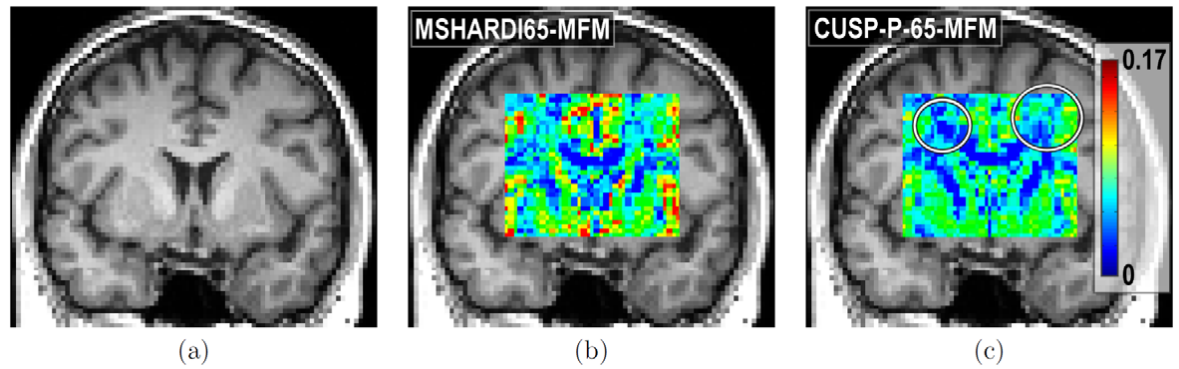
Comparison of CUSP and multi-shell HARDI via residual bootstrapping. (a) T1-weighted image showing the anatomy. (b) Standard deviation of the maximum FA when using MSHARDI-65-MFM. (c) Standard deviation of the maximum FA when using CUSP-65-MFM. The standard deviation of the maximum FA is significantly lower when using CUSP, showing a lower uncertainty in the MFM estimates.
